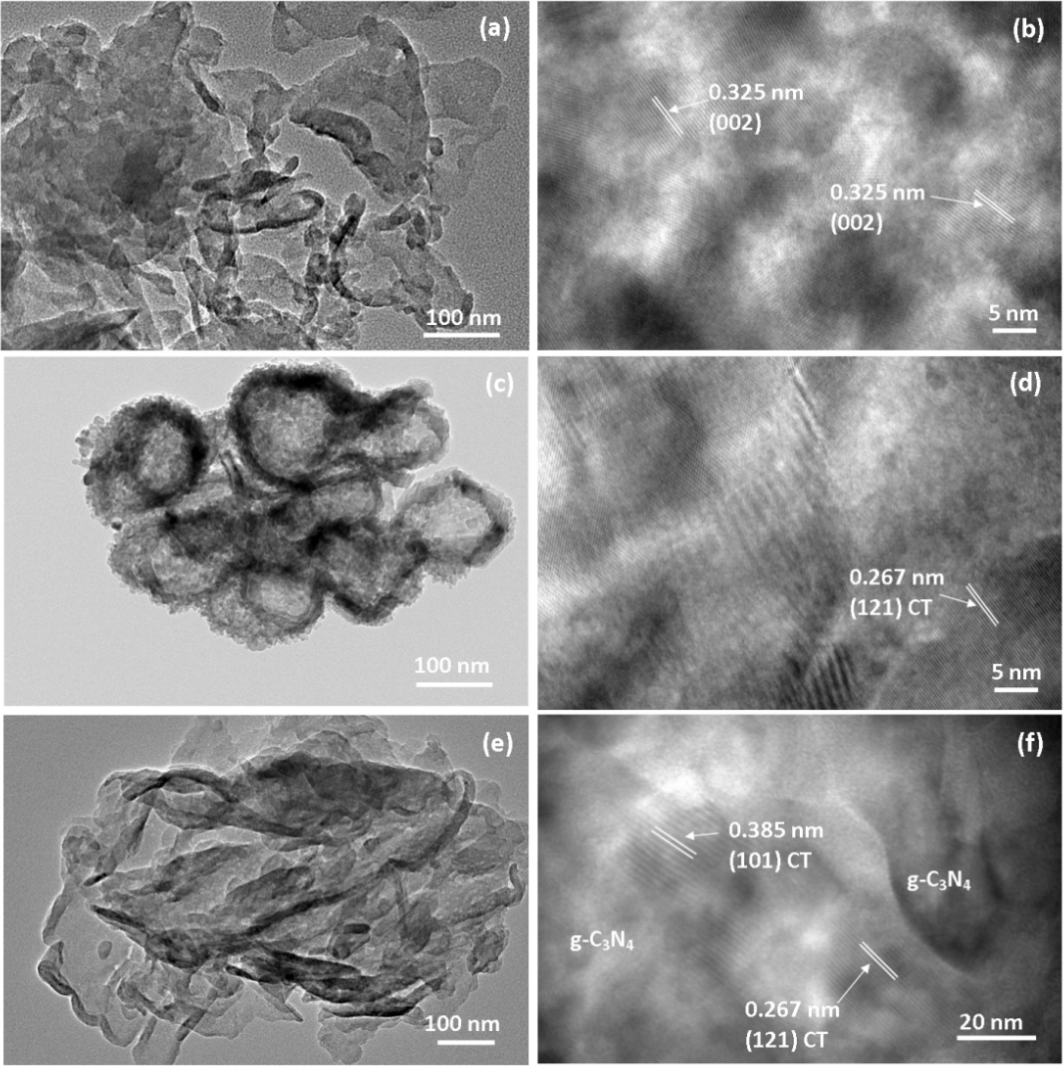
TEM images of (a, b) g-C_3_N_4_ nanosheets, (c, d) CT flakes and (e, f) CTCN heterojunction.
